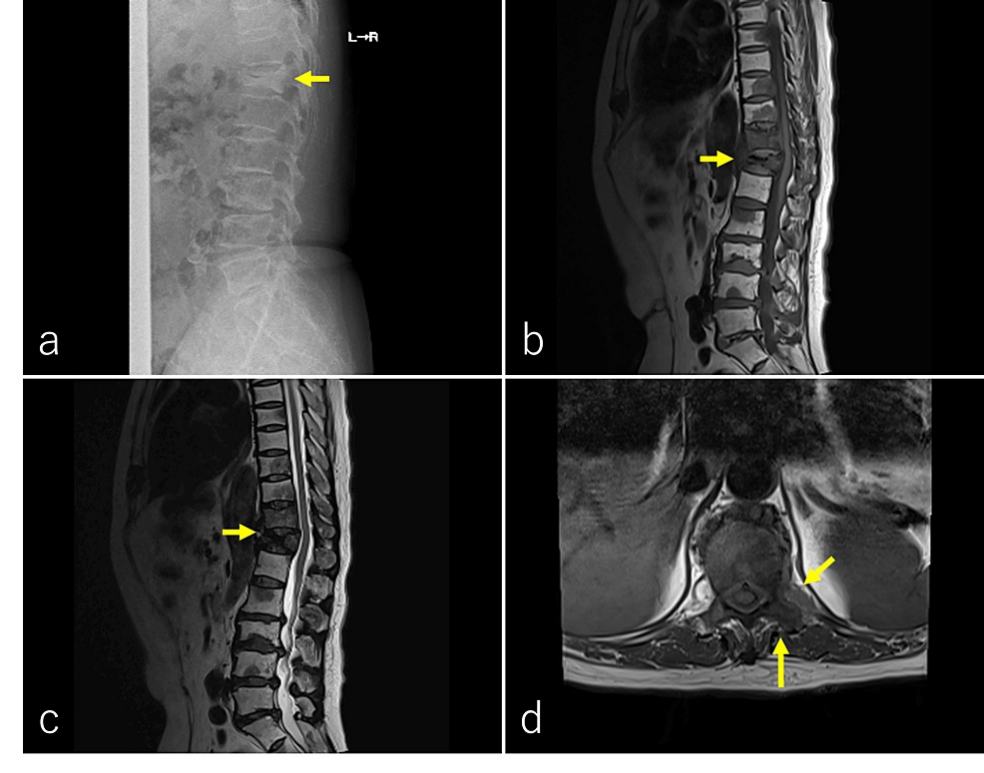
X-ray examination and vertebral magnetic resonance imaging. An X-ray examination revealed sclerotic changes and compression fracture of the 12th thoracic lumbar vertebra (a, yellow arrow). On the magnetic resonance imaging, a T1-weighted image showed low-signal findings in the 11th and 12th thoracic lumbar vertebrae and other lumbar vertebrae (b, yellow arrow). A T2-weighted image showed low-signal findings in the 11th and 12th thoracic lumbar vertebrae and other lumbar vertebrae (c, yellow arrow). A T1-weighted image showed tumor invasion on the left side from the 11th thoracic vertebra above the 1st lumbar vertebra (d, yellow arrows).
